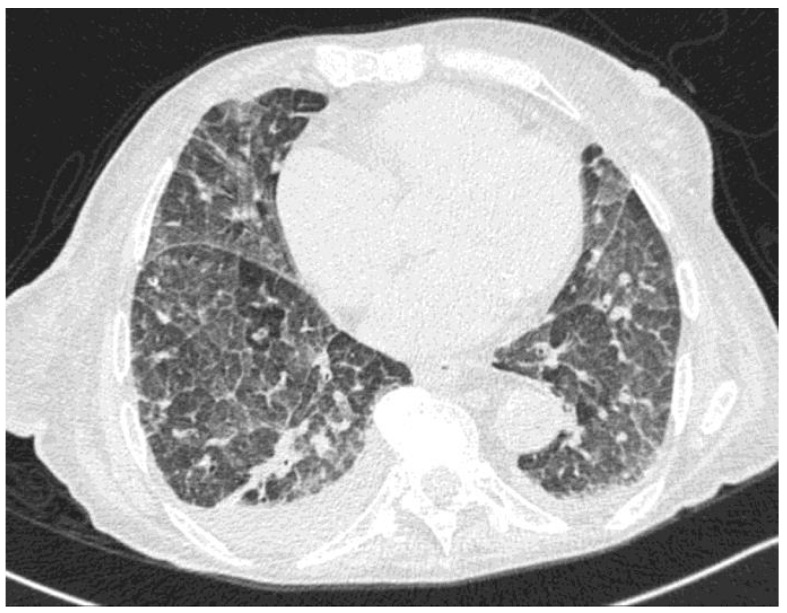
Chest CT showing diffuse ground-glass pattern and septal thickening.
